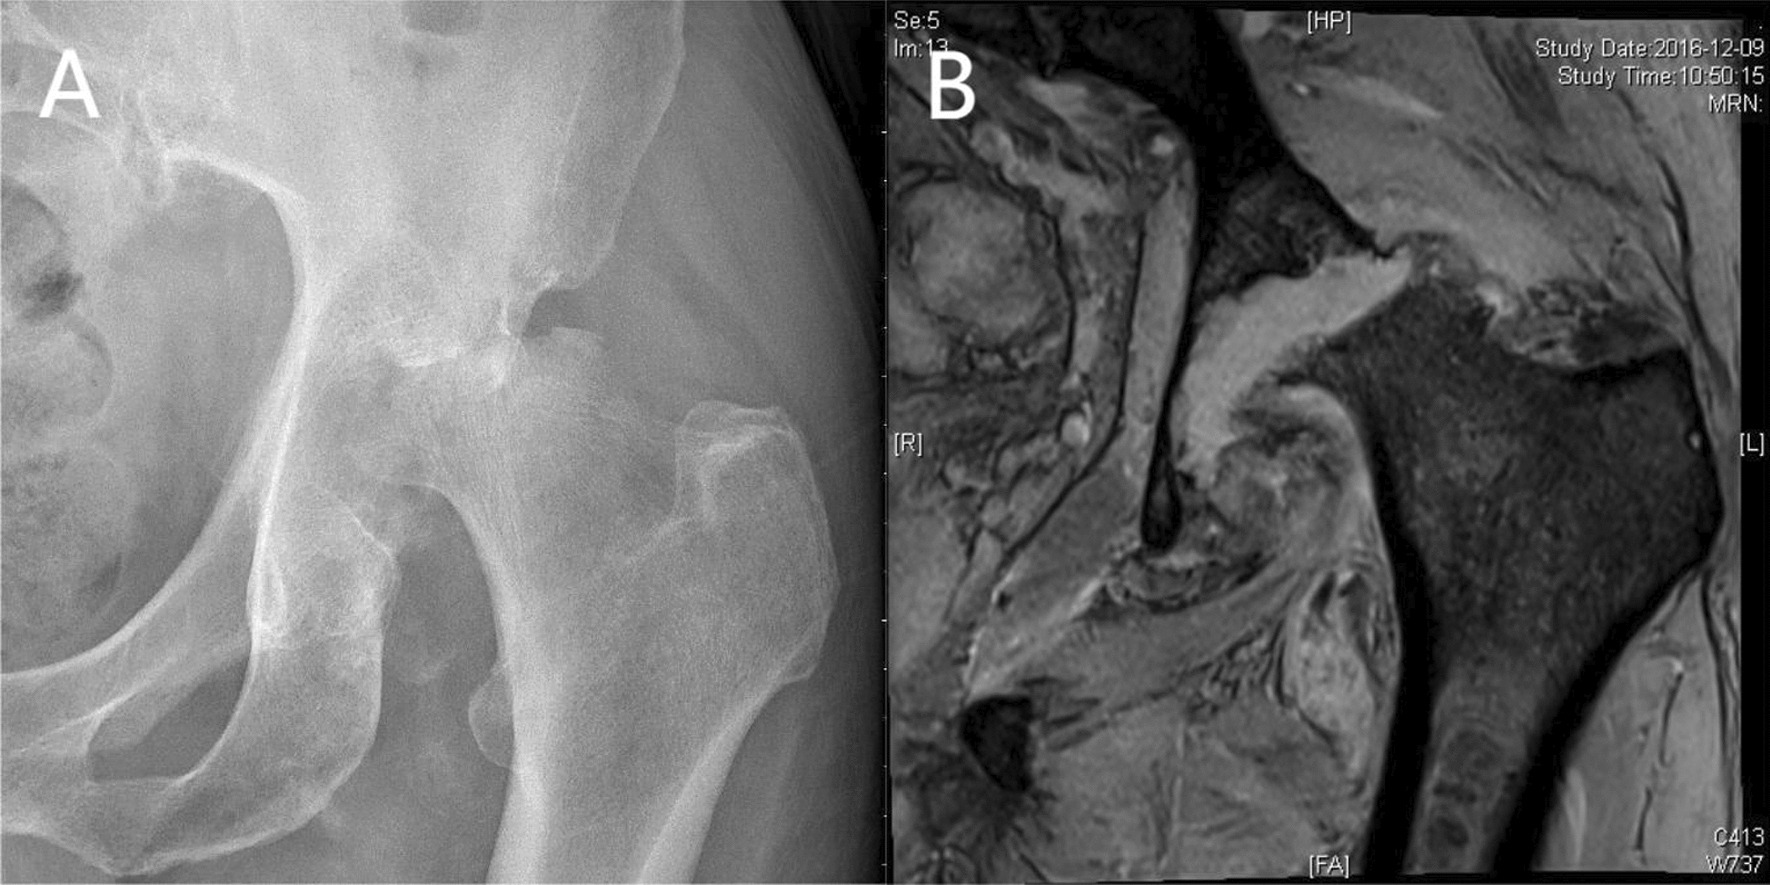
A 67-year-old male patient suffered from ONFH accompanied with septic hip (case 6). A The initial radiograph revealing the collapse of femoral head and narrowing of joint space. B MRI shows a large amount of effusion and synovial thickening of the hip
